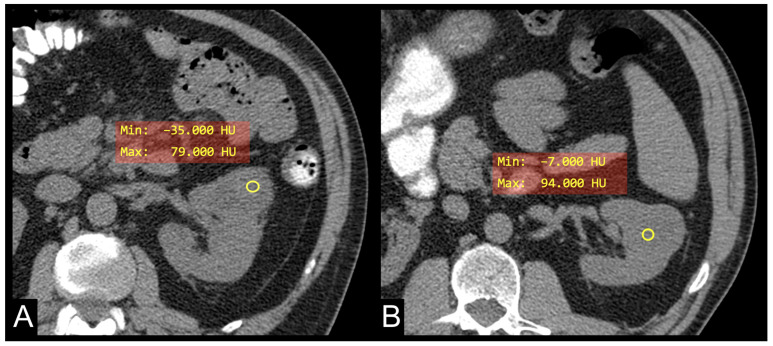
Unenhanced axial CT images of patients with low WHO/ISUP grade ccRCC with ADFP expression (A) and low WHO/ISUP grade ccRCC without ADFP expression (B) show yellow ROIs with different minimum tumor HU values (HU −35 and −7, respectively).
